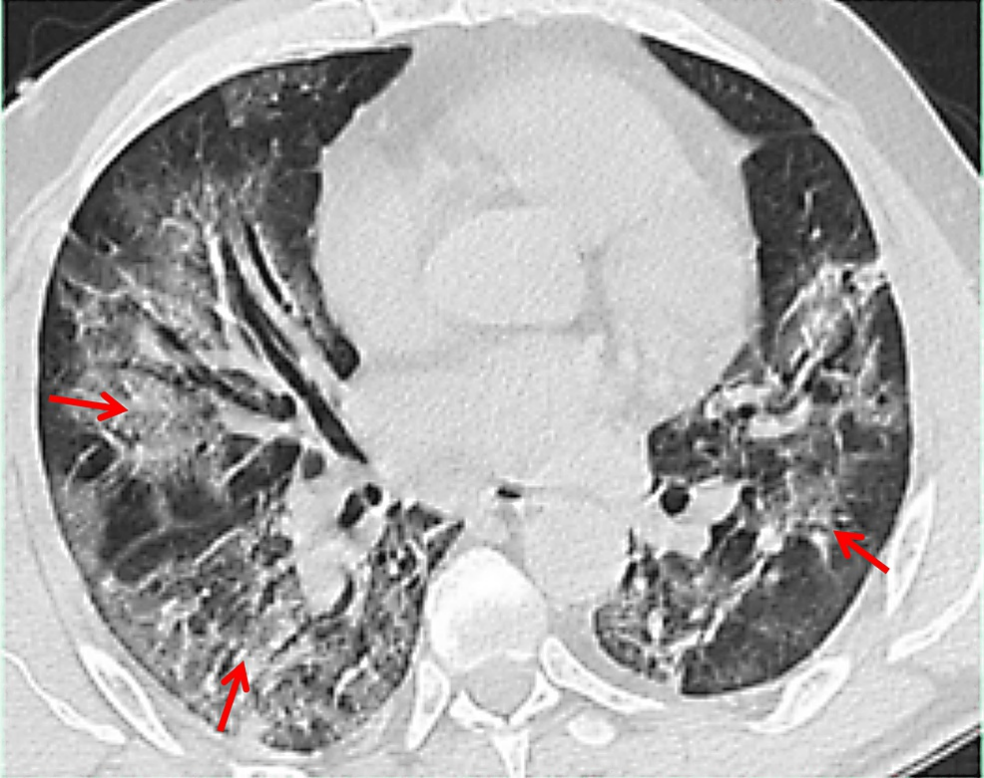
Axial chest CT scan with a parenchymal window shows a large ground glass and thickening of the bronchovascular axes (the arrows indicate some areas of lesions).
